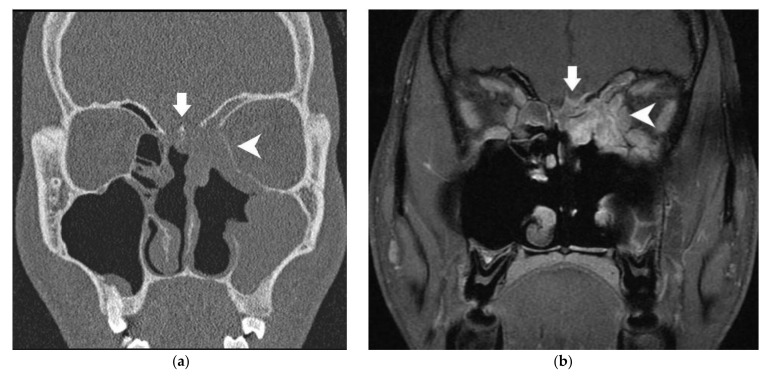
Twenty-year-old male subject with neuroendocrine carcinoma of the left ethmoid sinus. (a) Coronal CT scanner: minor erosion of the lamina papyracea (white arrowhead) and major erosion of the cribriform plate (white arrow). (b) Coronal MRI (T1 acquisition with fat saturation and gadolinium injection): white arrow: major modification of bony skull base, irregular nodular dural enhancement >2 mm, irregular deformation of the dura, contact angle ≤45°; white arrowhead: major modification of lamina papyracea, regular deformation of orbital content, invasion of the fat between tumor and oculomotor muscle. In the radiological conclusion, the bony skull base, orbital medial wall and dura were considered as invaded. Cerebral parenchyma and orbital content were considered as tumor-free.
